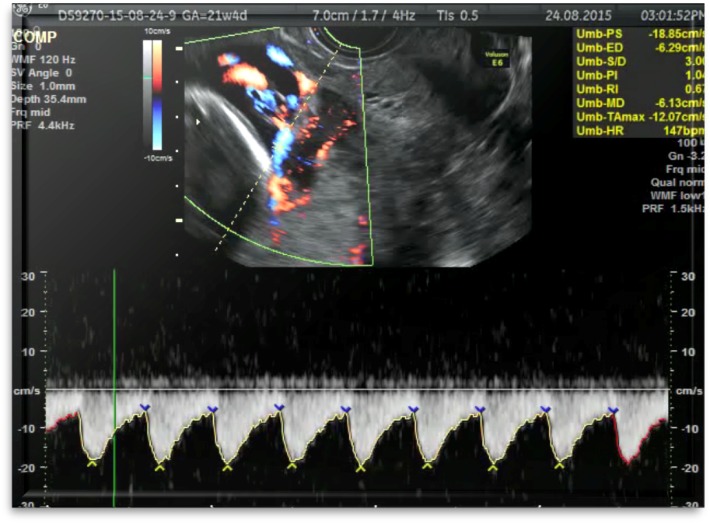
Case 2: pulsated Doppler transvaginal examination: Doppler spectrum characteristic for the vasa praevia umbilical artery
